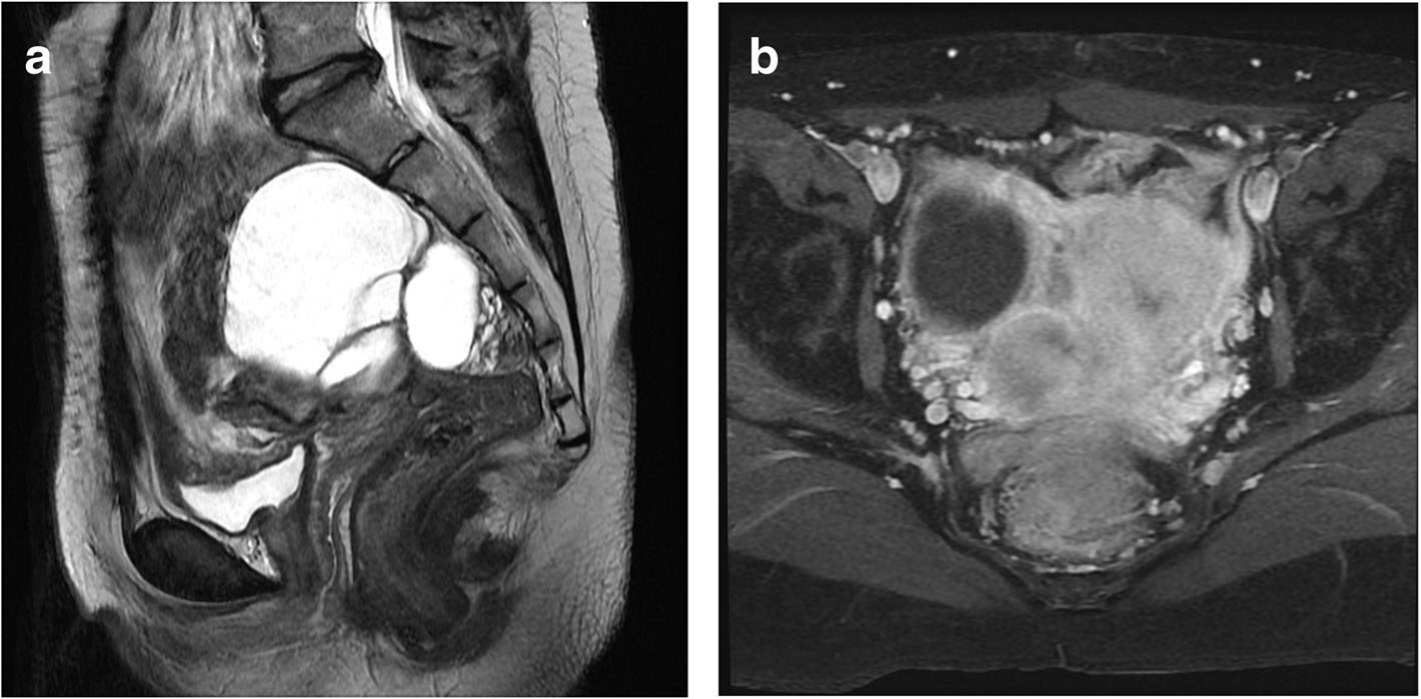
MRI T2-weighted images of pelvic cavity. a Sagittal view showing a 7.8 × 7.3-cm cystic mass with internal septa and blood content at right adnexa. b Axial view showing some enhancing soft tissue at the cul-de-sac with restricted diffusion on diffusion-weighted imaging, in favor of tumor seedings
